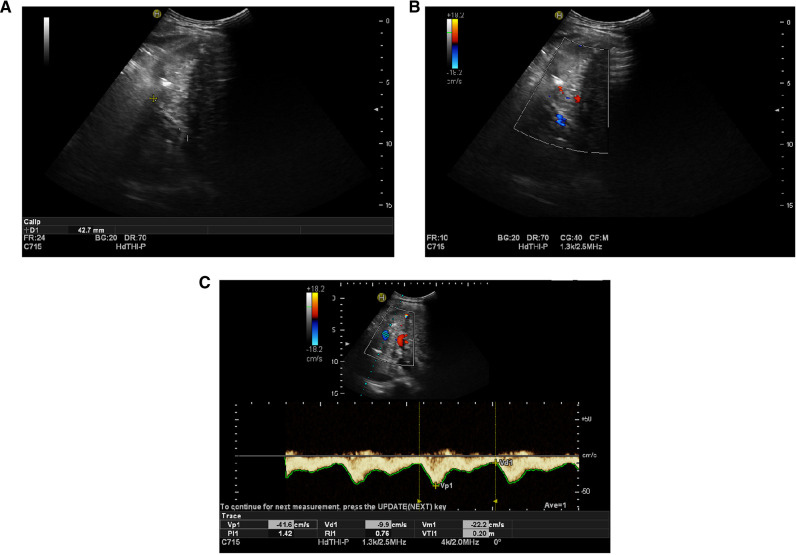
(A) Artificial vessels, which were visualized by ultrasound one week after operation, were approximately 42.7 mm in length. (B) Blood flow of artificial blood vessels displayed by ultrasound one week after operation (blue). (C) Ultrasound blood flow spectrogram of artificial vessels one week after operation, showing blood flow patency.
